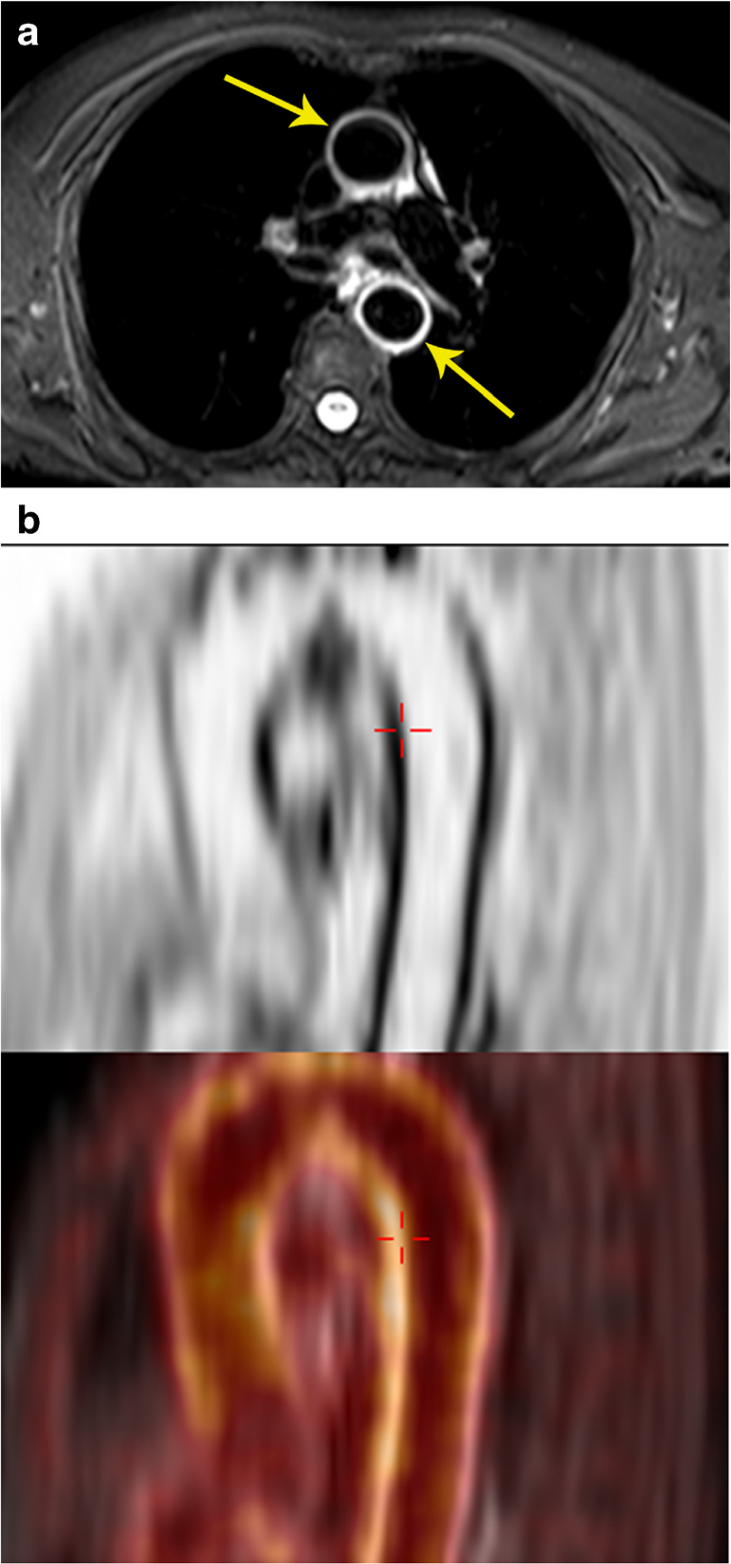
Vasculitis. a Axial STIR MRI image performed in a patient with suspected vasculitis following lung cancer chemotherapy shows an intense high signal in the wall of the ascending and descending aorta (arrows), which is indicative of active vasculitis. b Sagittal FDG PET image in the same patient shows the intense uptake along the wall of the thoracic aorta (arrow). c This high uptake is localized better to the aortic wall (arrow) using the fused PET/MR image (bottom)
